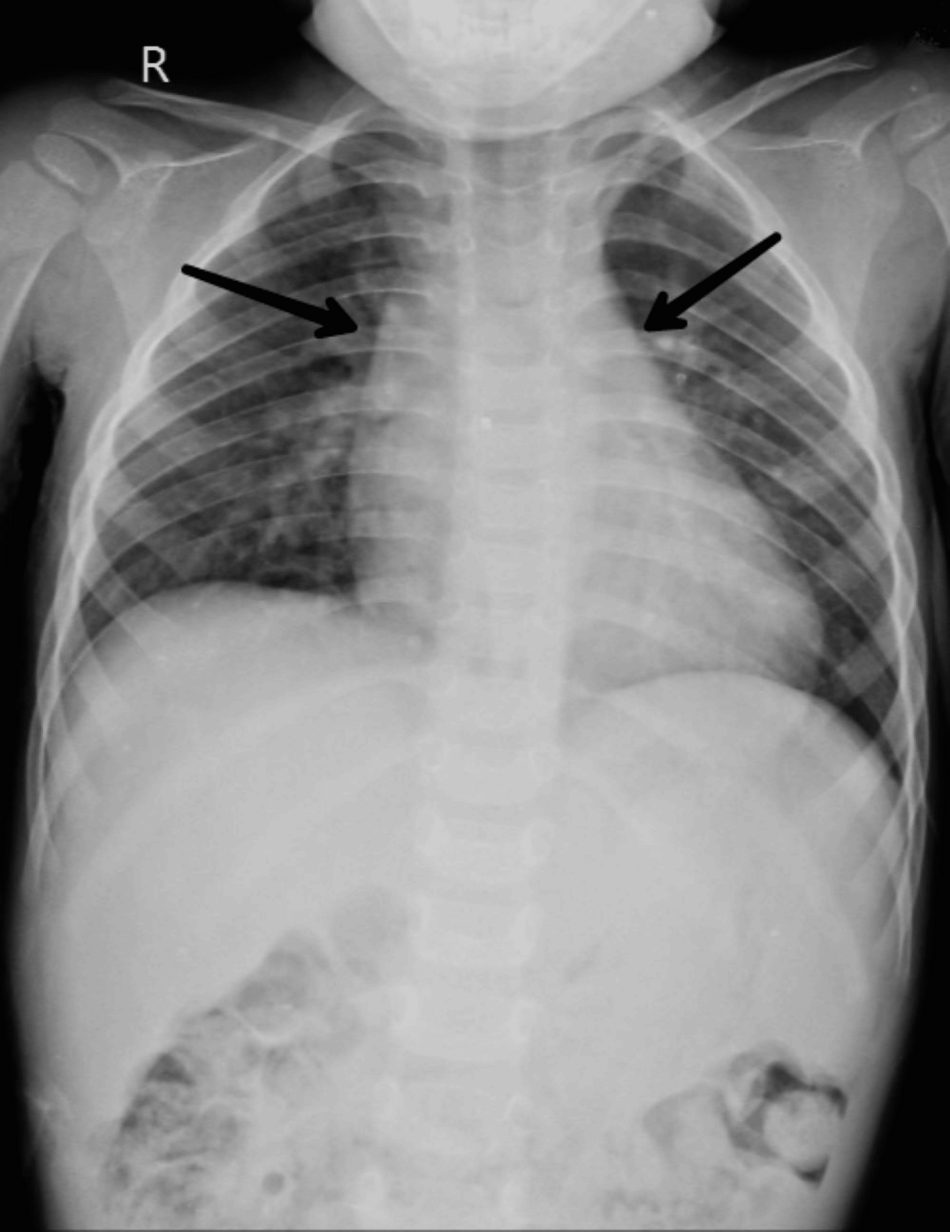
Preoperative AP chest radiograph AP: Anteroposterior
